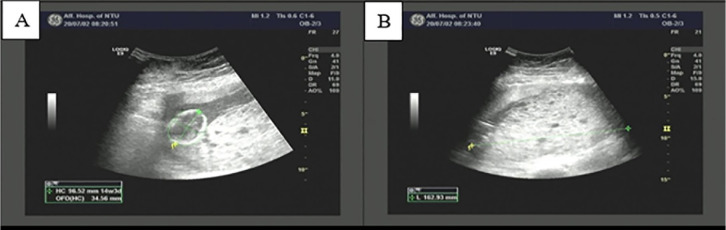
B-ultrasonography at 20 weeks of gestation showing intrauterine singleton
